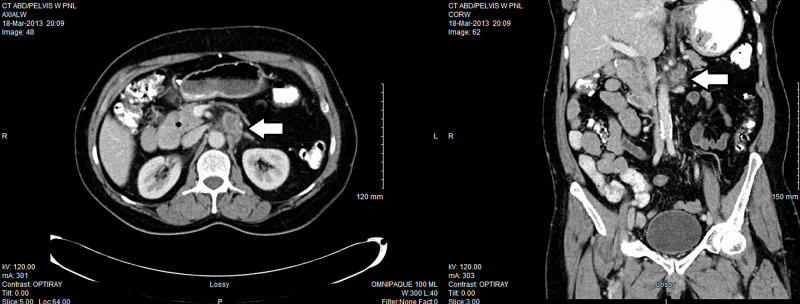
CT scan in March 2013 showing a new large retroperitoneal lymph node (white arrow) abutting the aorta, SMA and left renal vein. SMA, superior mesentery artery
